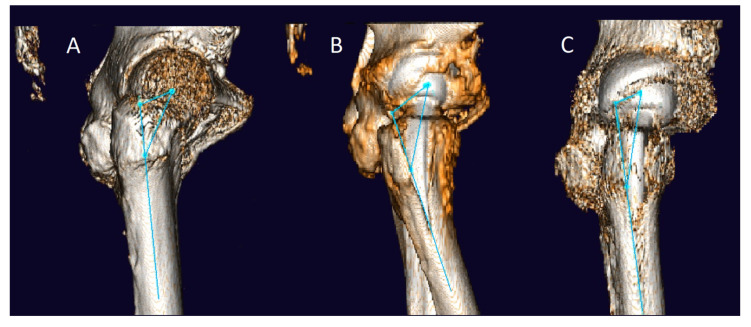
Preoperative and postoperative CT measurement method. 3D measurements obtained with mediCAD hip 3D software, showing the proximal femoral long axis defined by two points and the femoral anteversion angle formed between a perpendicular line through the proximal femoral long axis and the femoral head, and a line connecting the femoral condyles. (A): preoperative condition. (B): postoperative condition with short stem. (C): postoperative condition with straight stem.
